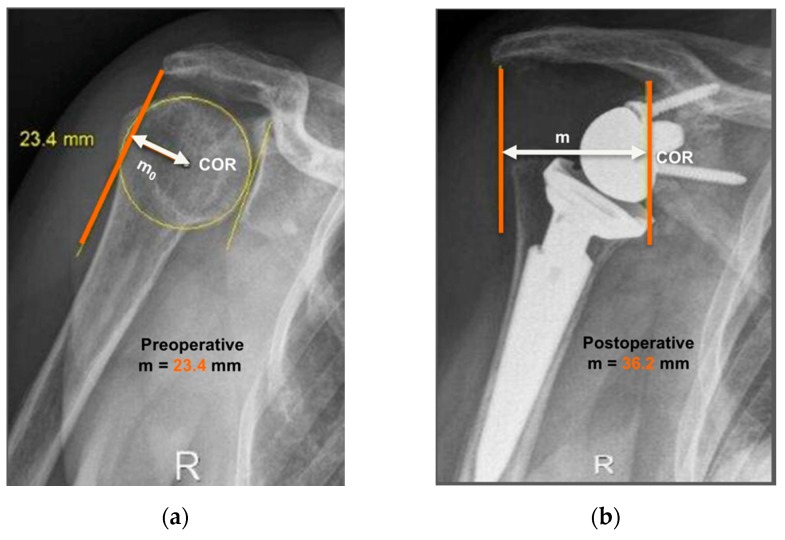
Anteroposterior radiographs of the same patient pre- and post-operatively are shown. Evaluation of center-of-rotation (COR) medialization was performed according to the protocol of Greiner et al. [5]. Pre-operatively (a), the distance “m_o_” was measured between the center of the circle involving the articular surface (COR) and the vertical line from the outer border of the acromion. Post-operatively (b), the distance “m” was measured between the center of the base plate (COR) and the vertical line from the outer border of the acromion. COR medialization was calculated as the difference between the distances “m_o_” and “m” as follows: ∆m = m_o_ − m. m_o_ > m was found in all subjects (12.8 mm for this representative patient).
